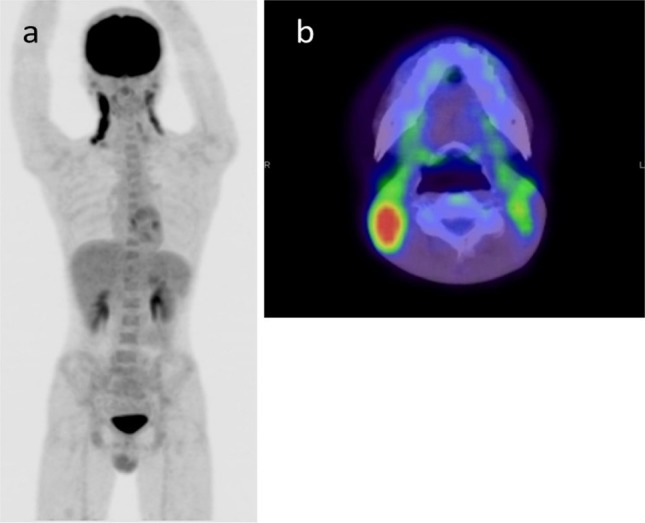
A 21-year-old man with Kikuchi’s disease misdiagnosed as lymphoma; (a) Maximum intensity projection image and (b) fused FDG-PET/CT image of the neck are shown. The patient presented with cervical lymphadenopathy and a fever of 39°C for a month. FDG-PET/CT showed bilateral cervical lymphadenopathy with elevated FDG uptake (SUV_max_=8.7). Elevated sIL-2R of 1200 U/ml was also observed. Although the evaluators commented that this case was compatible with lymphoma (degree of suspicion was Grade 3), the final biopsy-proven diagnosis was Kikuchi’s disease
